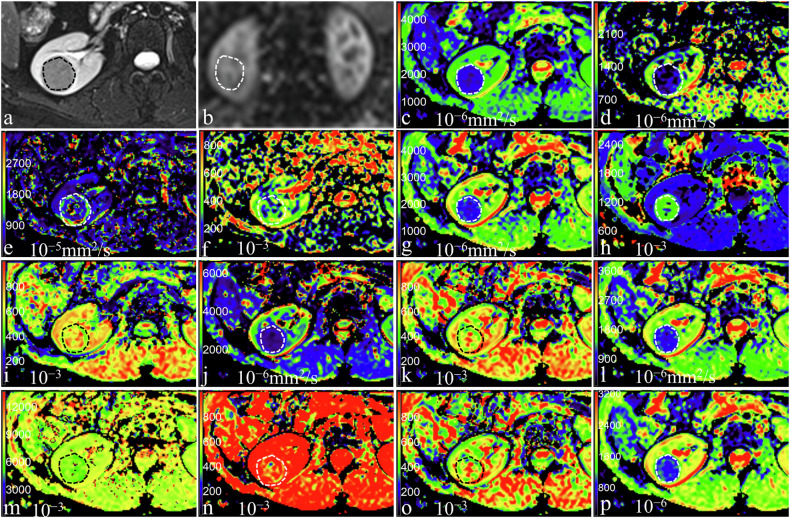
A 26-year-old woman with pathologically confirmed fpAML in the right kidney. a Axial T2-weighted imaging shows a low-signal-intensity mass; b RBF map shows the perfusion level of mass, with the RBFmean and RBFpeak of 190.56 mL/100 g/min and 244.00 mL/100 g/min; c–p pseudo-colorized images showed the Mono_ADC (c), IVIM_D (d), IVIM_D* (e), IVIM_f (f), DKI_MD (g), DKI_MK (h), SEM_α (i), SEM_DDC (j), FROC_β (k), FROC_D (l), FROC_μ (m), CTRW_α (n), CTRW_β (o), and CTRW_D (p), with the values of 1.07 × 10^−3^ mm^2^/s, 0.69 × 10^−3^ mm^2^/s, 11.56 × 10^−3^ mm^2^/s, 23.89%, 1.44 × 10^−3^ mm^2^/s, 0.98 × 10^−3^, 0.58 × 10^−3^, 0.97 × 10^−3^ mm^2^/s, 0.64 × 10^−3^, 1.04 × 10^−3^ mm^2^/s, 6.58 × 10^−3^, 0.88 × 10^−3^, 0.63 × 10^−3^, and 1.01 × 10^−3^ mm^2^/s, respectively
